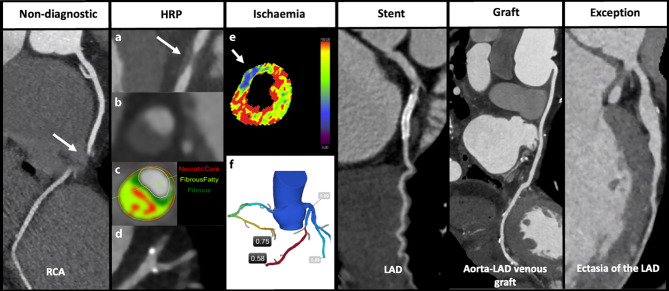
CAD-RADS modifiers: a low-attenuation plaque; b plaque with positive remodelling and napkin-ring sign; c semi-automated assessment of plaque composition; d spotty calcifications (< 3 mm); e computed tomography perfusion image with perfusion defect (white arrow) in the territory of LAD; f fractional-flow reserve (FFR) CT with haemodynamic significant stenosis in the LAD and RCA. *HRP* high-risk plaque*, LAD* left anterior descending, *RCA* right coronary artery
